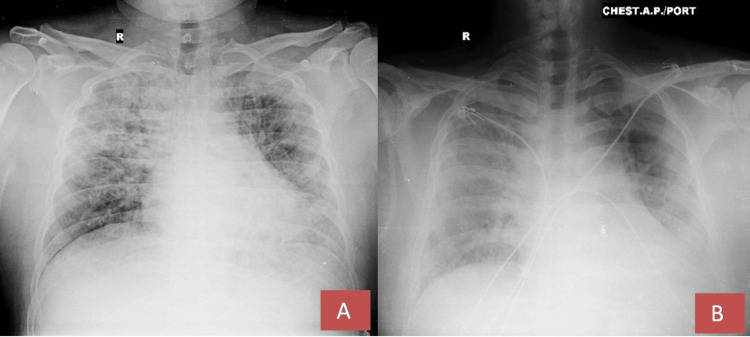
Chest X-ray findings A portable chest X-ray taken during the first week (A) and the second week (B) indicates a reduction in the radiological signs of pulmonary edema compared to the X-ray taken at the time of presentation.
